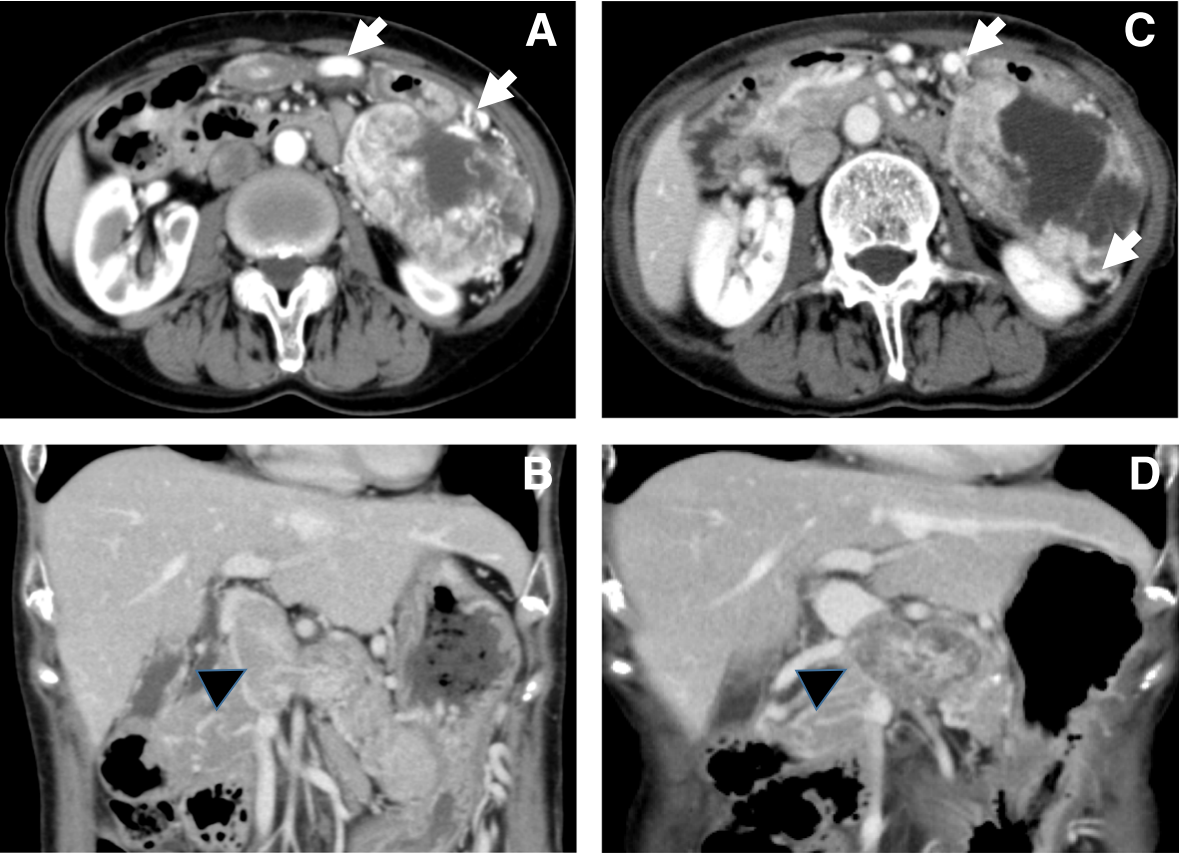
Abdominal computed tomography image showing a huge tumor in the left upper abdomen. Images were collected a, b at the time of initial diagnosis and c, d after everolimus therapy. The *arrows* indicate the collateral vessels, and the *arrowhead* indicates a tumor invasion into the portal vein
